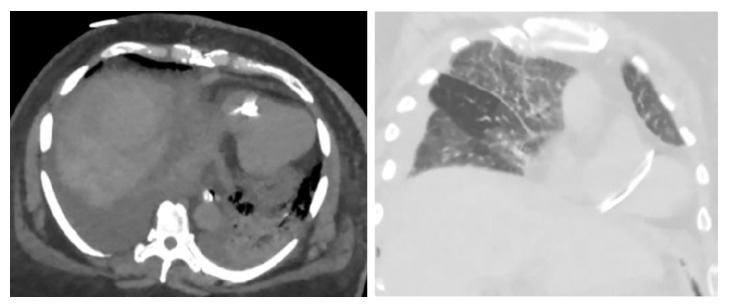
CT chest showing the fractured catheter in the right ventricle.
